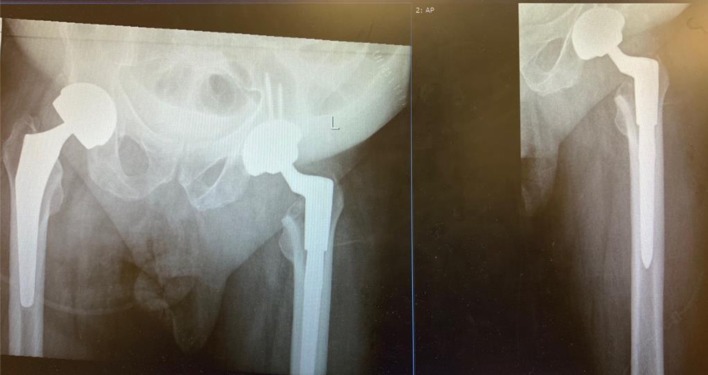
AP pelvis and left hip post-operative check X-rays.
